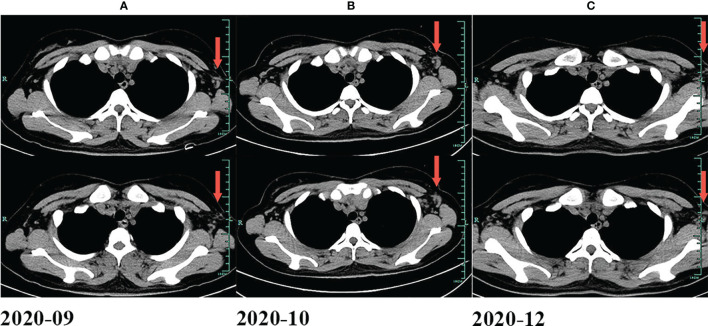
Changes of lymph nodes evaluated by CT. (A) In September 2020. (B) In October 2020. (C) In December 2020.
